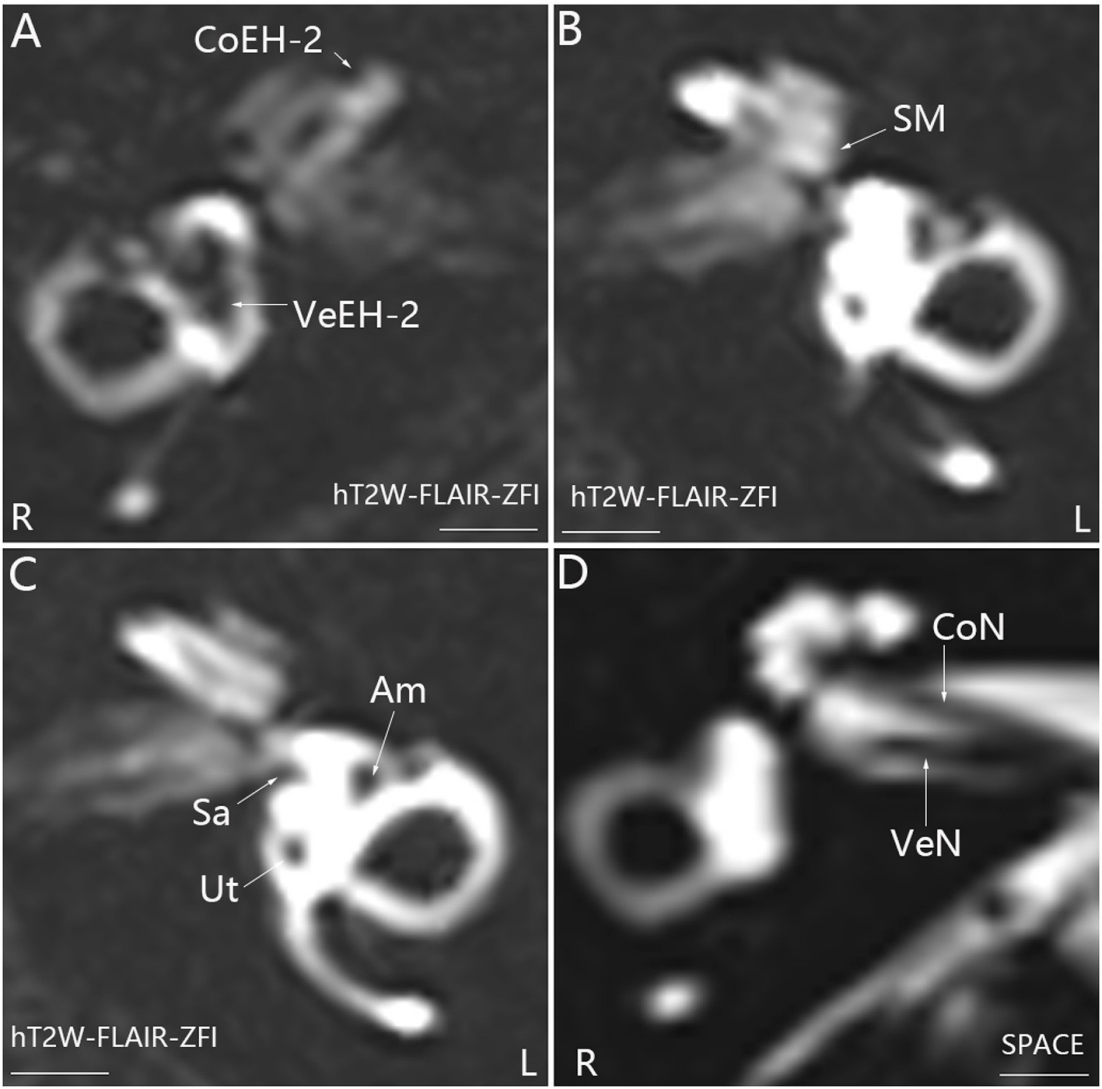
Representative MRI demonstrating EH in the right ear (R) in patient *P* with definite MD on the right side (A). MR images were taken using the hT_2_W-FLAIR-ZFI sequence 24 h after intratympanic injection of 0.1 ml of 20-fold-diluted Gd-DTPA that was delivered onto the posterior upper part of the tympanic medial wall on both sides. There was no EH in the left ear (L) (B, C). Neither inner ear fibrosis nor vestibular schwannoma was found on the ipsilateral side imaged using the SPACE sequence (D). Am, ampulla; CoEH-2, grade 2 cochlear EH; CoN, cochlear nerve; Sa, saccule; Ut, utricle; VeEH-2, grade 2 vestibular EH; VeN, vestibular nerve. Scale bar = 3.0 mm.
